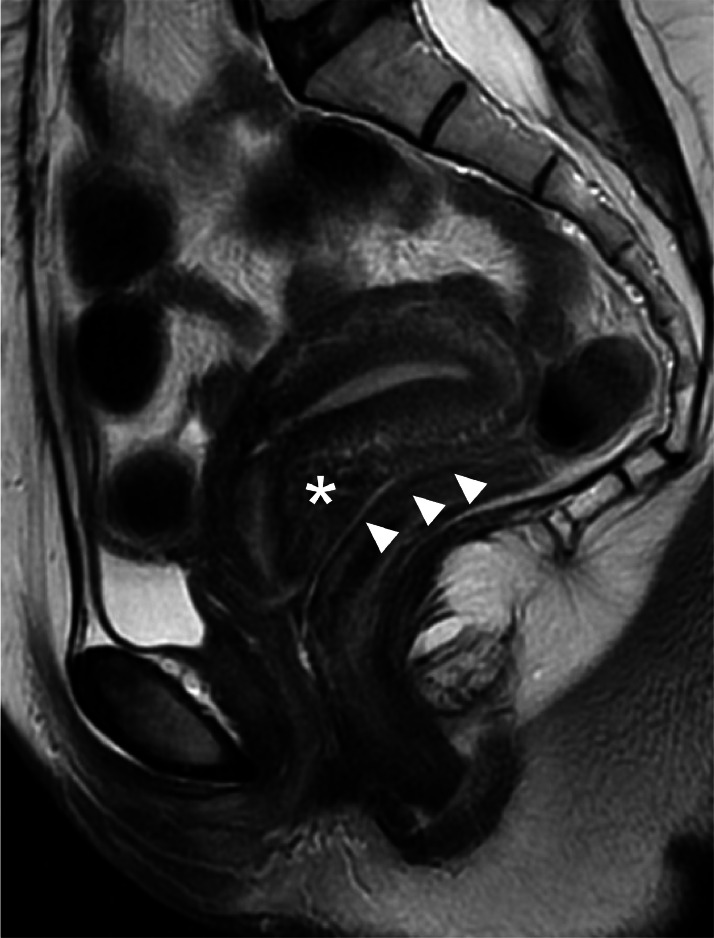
Prepregnancy magnetic resonance imaging Sagittal T2-weighted image showing a retroflexed uterus. The elevated posterior vaginal fornix (*) and the absence of free peritoneal fluid in the Douglas pouch (*arrowhead*) suggest posterior cul-de-sac obliteration associated with severe endometriosis.
